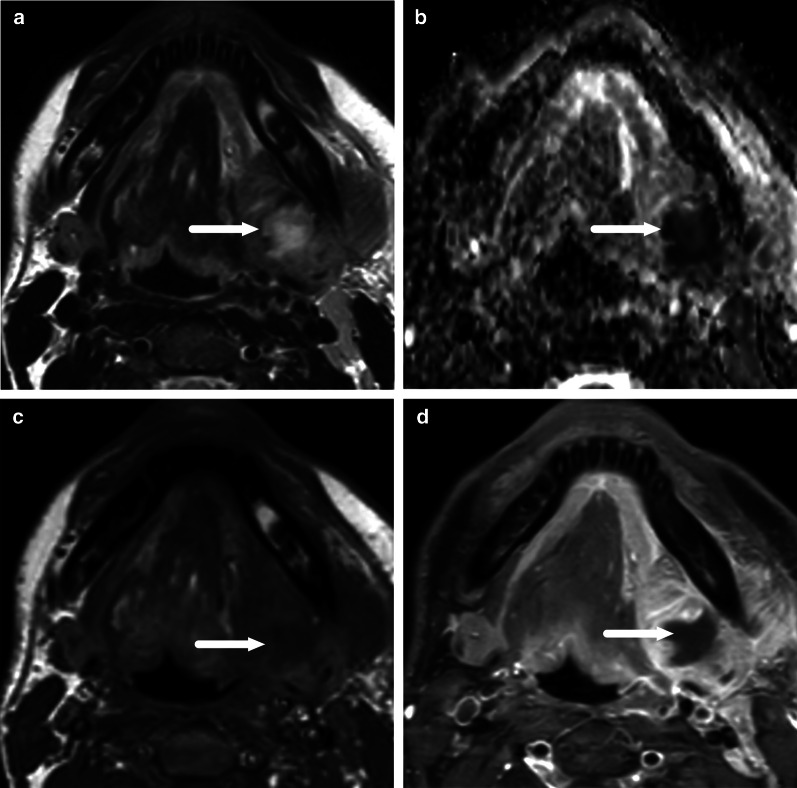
Diagnostic criteria for an abscess on MRI: axial T2-weighted (a), pre-contrast T1-weighted (c), fat-suppressed post-contrast T1-weighted (d) images, and an ADC map (b). A submandibular space odontogenic abscess (arrows) shows intermediate to high T2-signal (a), no enhancement after contrast injection (c, d), and restricted diffusion as indicated by low ADC values (b)
